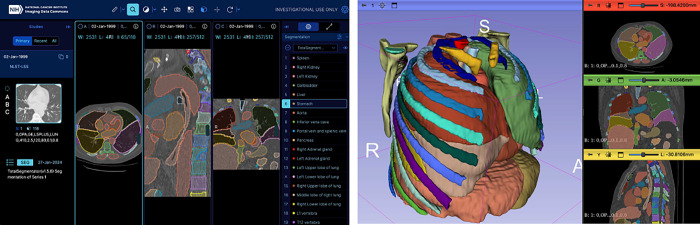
Visualization of a sample CT series and the corresponding segmentation. The case shown is for NLST PatientID 100004, time point 0. Left: Visualization using OHIF v3 viewer integrated within IDC Portal, as can be accessed at https://viewer.imaging.datacommons.cancer.gov/v3/viewer/?StudyInstanceUIDs=1.2.840.113654.2.55.174144834924218414213677353968537663991&SeriesInstanceUIDs=1.2.840.113654.2.55.195946682403058845904768502826466194287,1.2.276.0.7230010.3.1.3.313263360.11967.1706318547.125050. Right: Visualization of the same CT and SEG series using 3D Slicer 5.6.2 (SlicerIDCBrowser extension was used to download data from IDC, QuantitativeReporting extension was installed to support loading of DICOM SEG into the Slicer scene).
